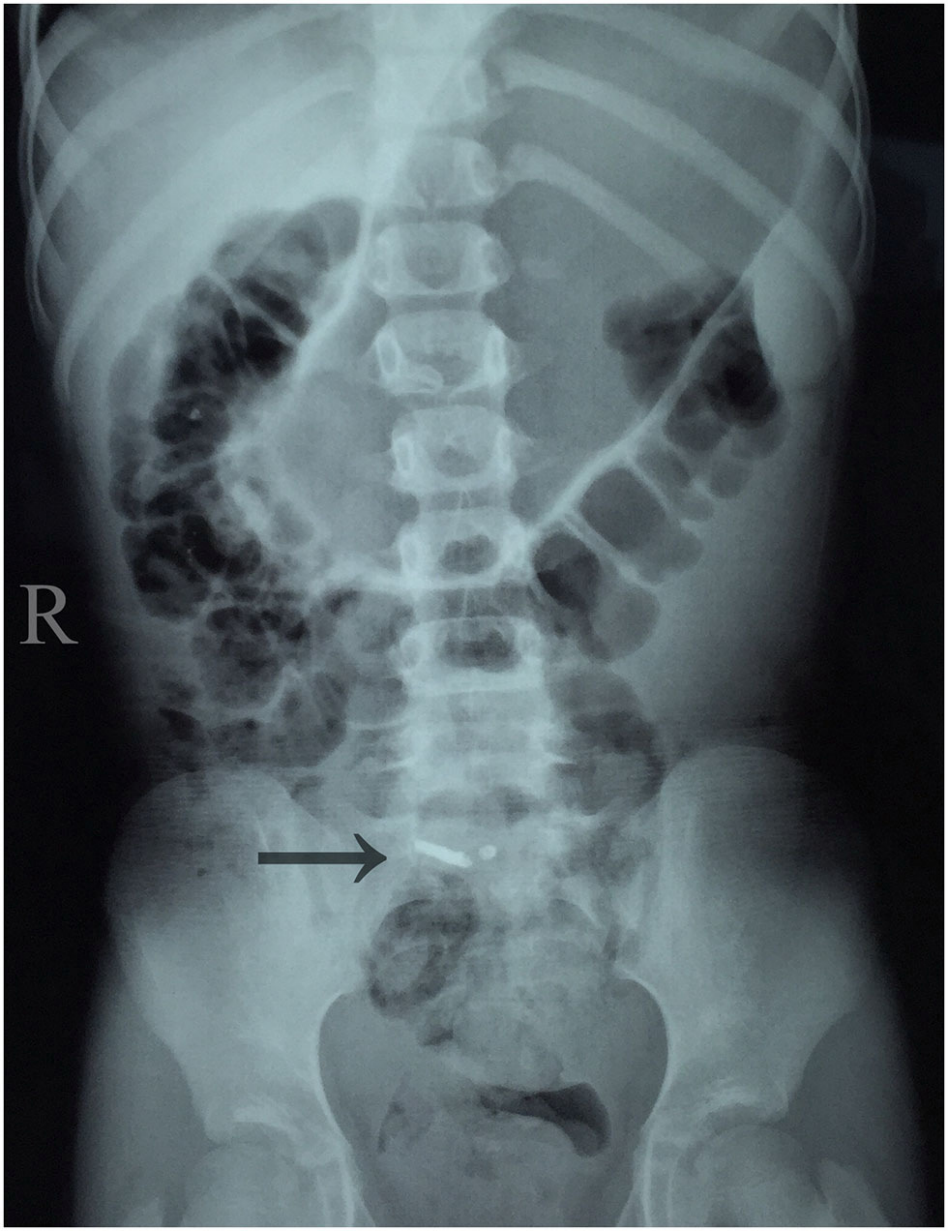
Abdominal plain film showing radiopaque tubular structure around the ileocecal junction in the right lower quadrant.
